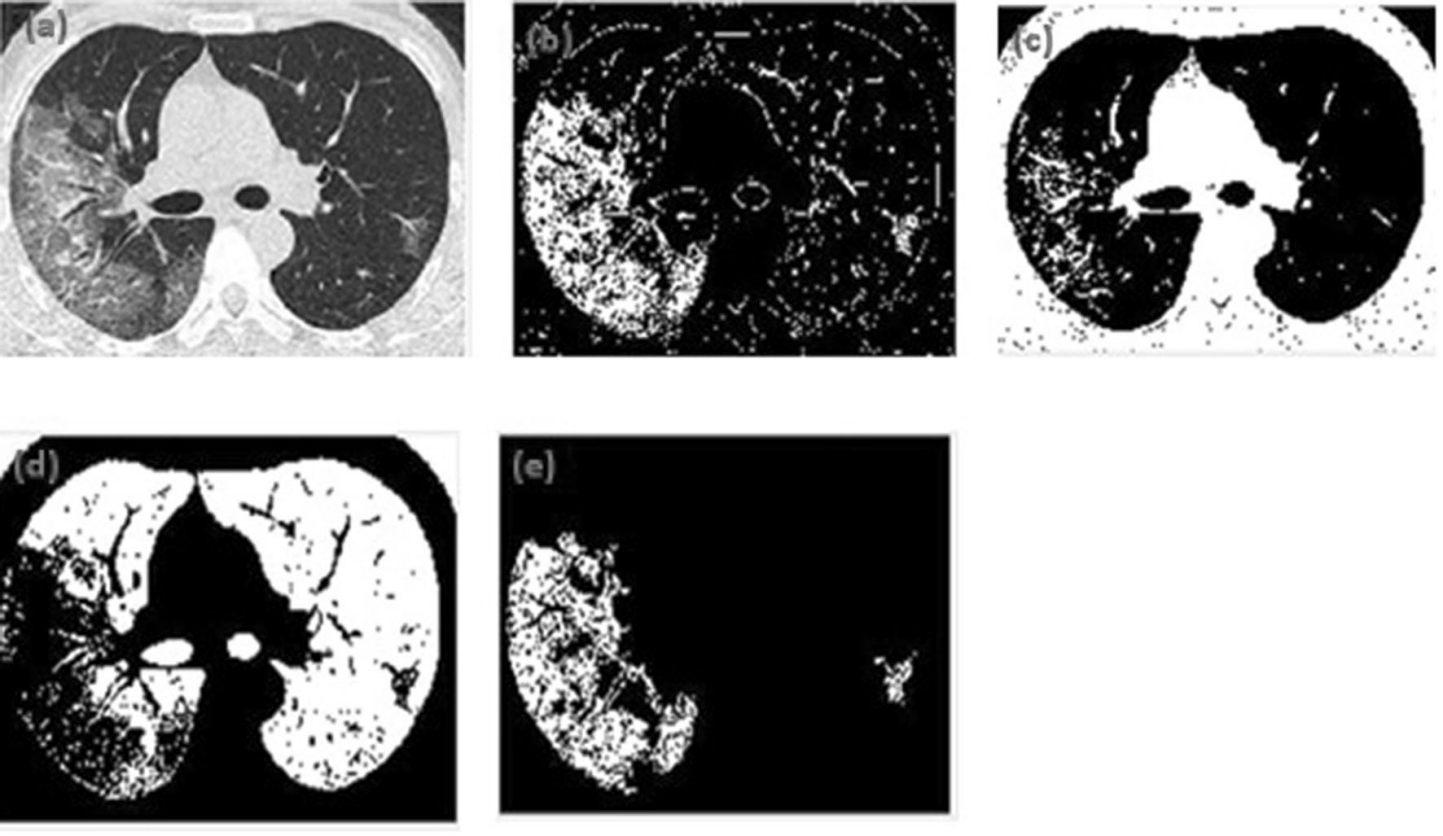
k*-means segmentation of COVID CT image (a) original CT image of COVID-19 patient, (b) cluster depicting the region of interest, (c) cluster that detects several edges in the image, including the lungs, vessels, and some of the ground glass opacities, (d) cluster depicting uninfected regions of the lungs, (e) final *k*-means image highlighting lung infection such as ground-glass opacities.
